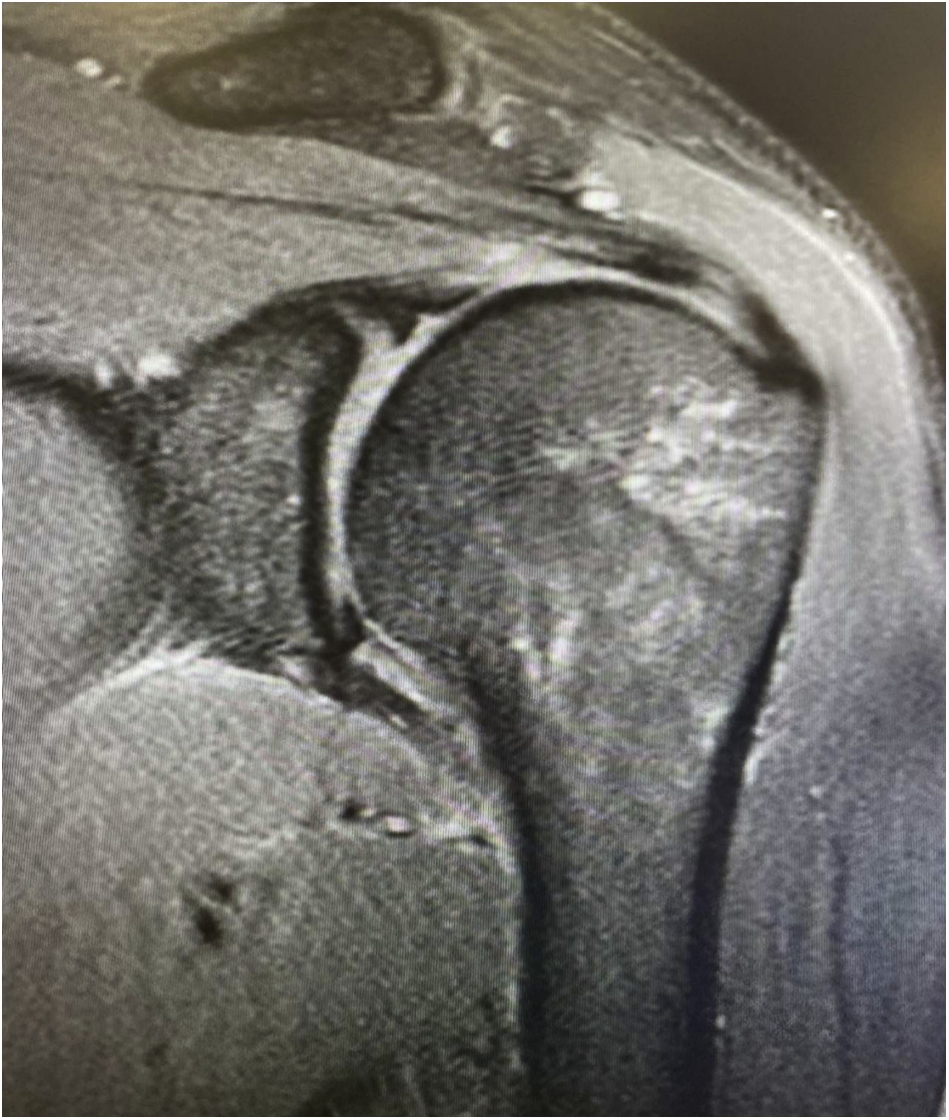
Our patient’s MRI significant for marginal tendonitis of the supraspinatus tendon with nonspecific subacromial edema. *MRI*, magnetic resonance imaging.
